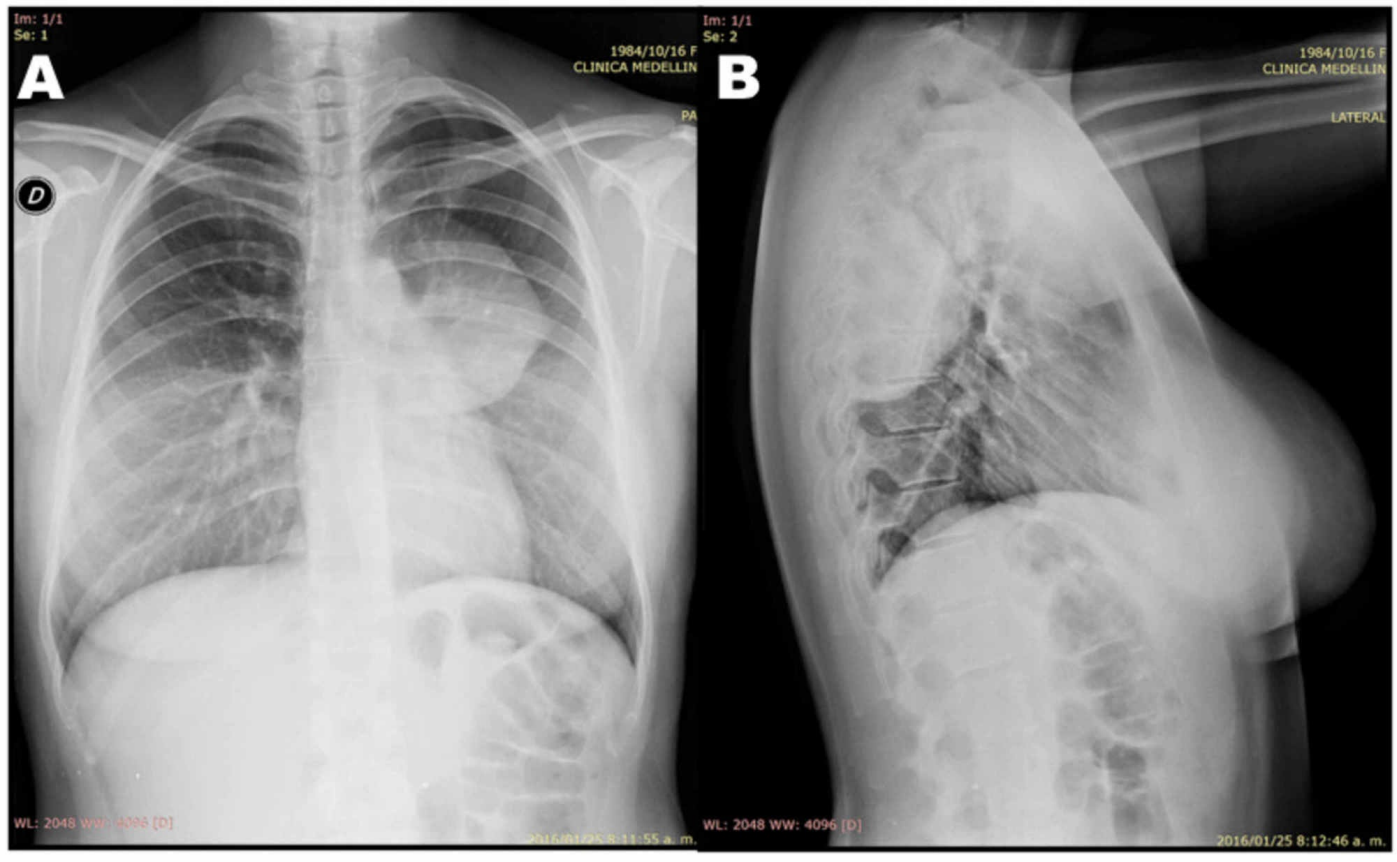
Posteroanterior and lateral CXR. Posteroanterior (A) and lateral (B) CXR showing a dense well-defined mass located on the left posterior mediastinum, with smooth borders, a minimal reaction in the adjacent lung tissue, acute angle borders, with no rib erosion of the overlying mass, or enlarged neural foramina. Breast implants are noted. CXR, chest X-ray
